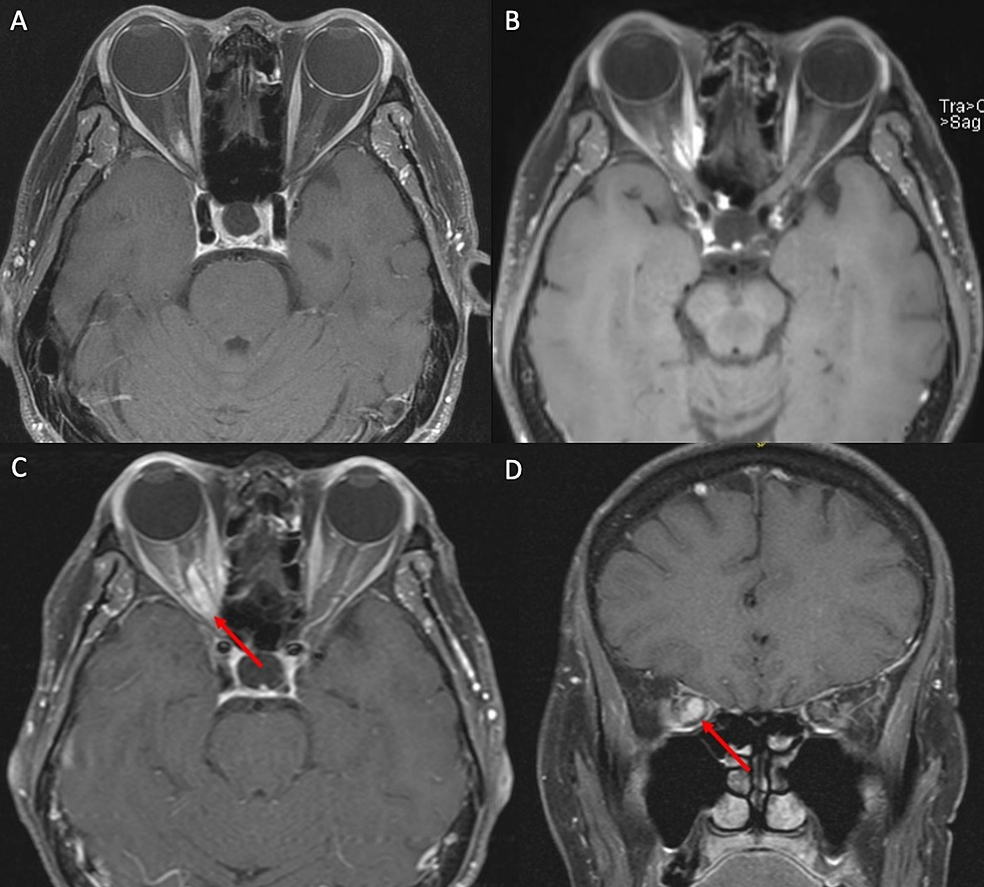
MRI demonstrating ONSM before and after fractionated radiotherapy. MRI demonstrated the tumor along the right optic nerve. (A) Initial axial T1 contrast-enhanced MRI upon presentation before radiotherapy demonstrated an enhancing lesion along the medial aspect of the optic nerve along the medial aspect of the orbital apex. (B) Five years after radiotherapy, the patient was noted to have stability on surveillance MRI without changes in vision. (C and D) Seven years after the initial presentation, the patient had 12 months of progressive vision loss with a more circumferential lesion (red arrow) prior to radiosurgery. MRI: magnetic resonance imaging; ONSM: optic nerve sheath meningioma
